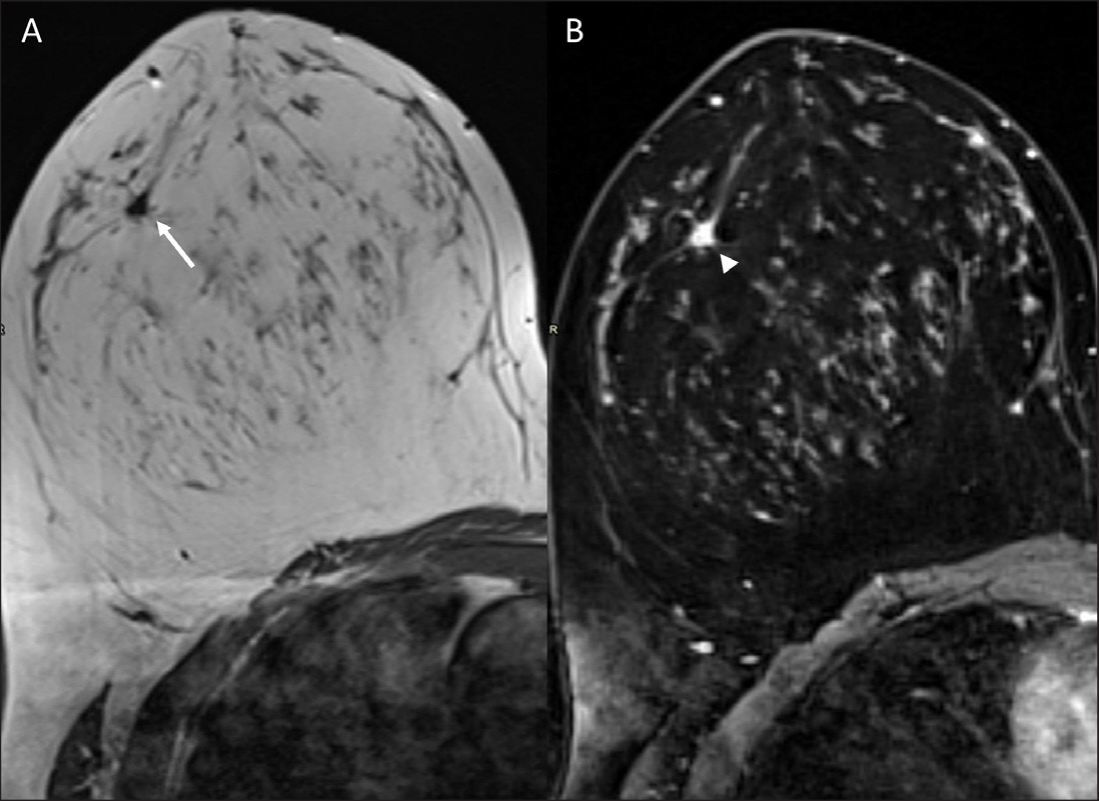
(A) Axial T1-weighted and (B) axial T1-weighted fat-suppressed contrast-enhanced MR images. Desmoid tumor superolateral in the right breast with irregular and stellate configuration (arrow) and enhancement (arrowhead).
